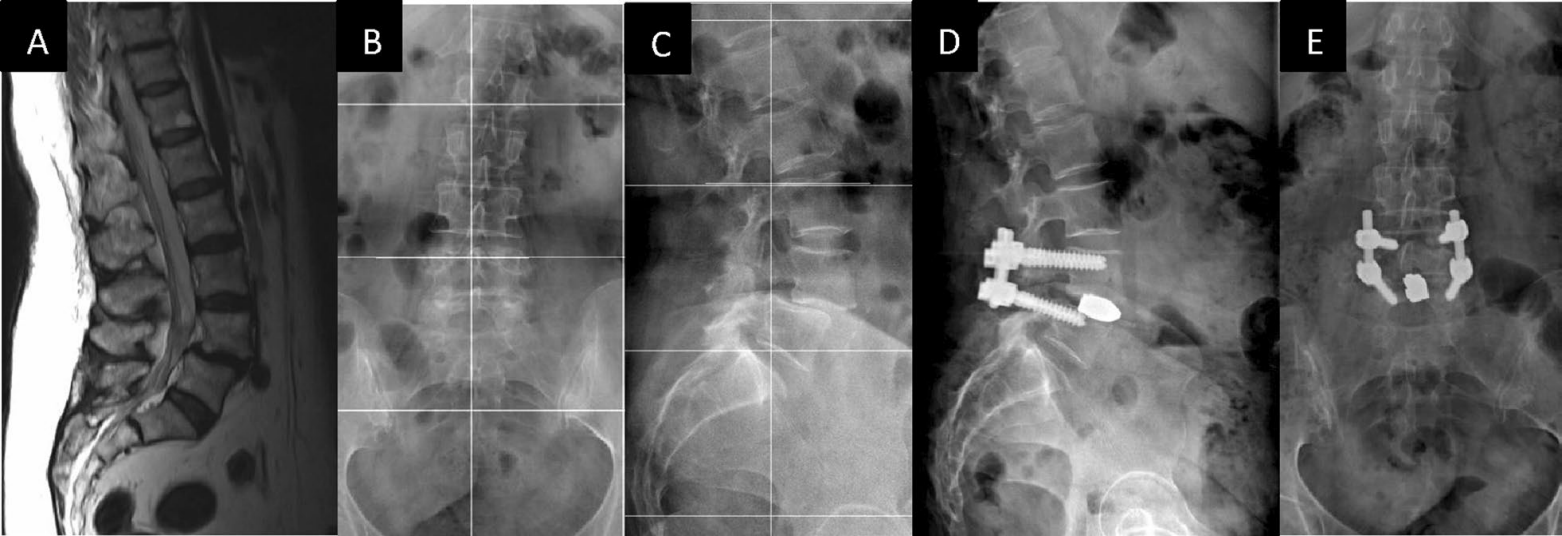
A Pre-op MRI with L4–L5 discopathy and spondylolisthesis. Lumbosacral spine X-rays: B pre-op AP view, C pre-op lateral view (L4–L5 lordosis 4°, L4–S1 lordosis 30°), D post-op lateral view (L4–L5 lordosis 18°, L4–S1 lordosis 38°), E post-op AP view
